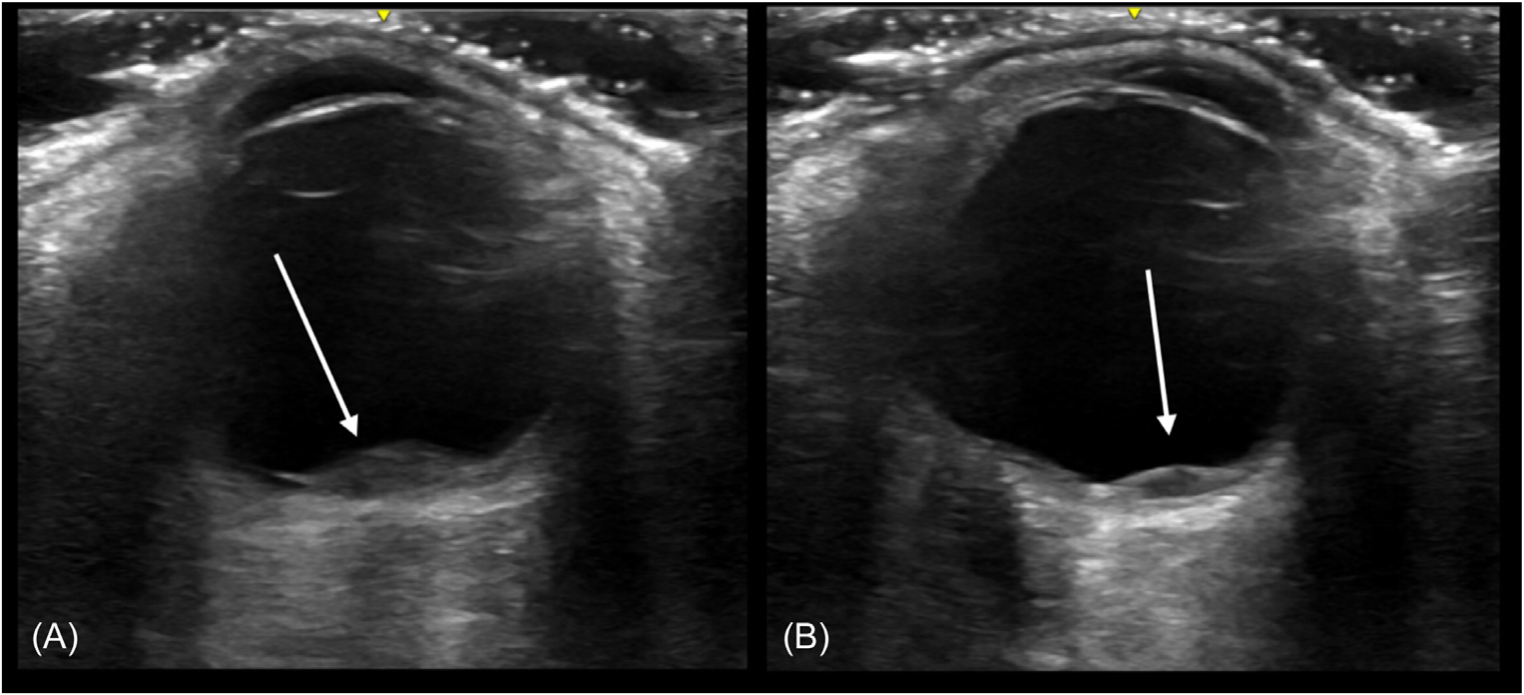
Point-of-care ultrasound using liner 8-megahertz transducer in transverse view demonstrating, (A) hypoechoic lenticular-shaped optic metastasis (3.2 millimeters [mm] by 1.5 mm) over the optic nerve, and (B) a smaller lenticular-shaped metastasis (1.7 mm by 1.0 mm) with the patient in leftward gaze.
